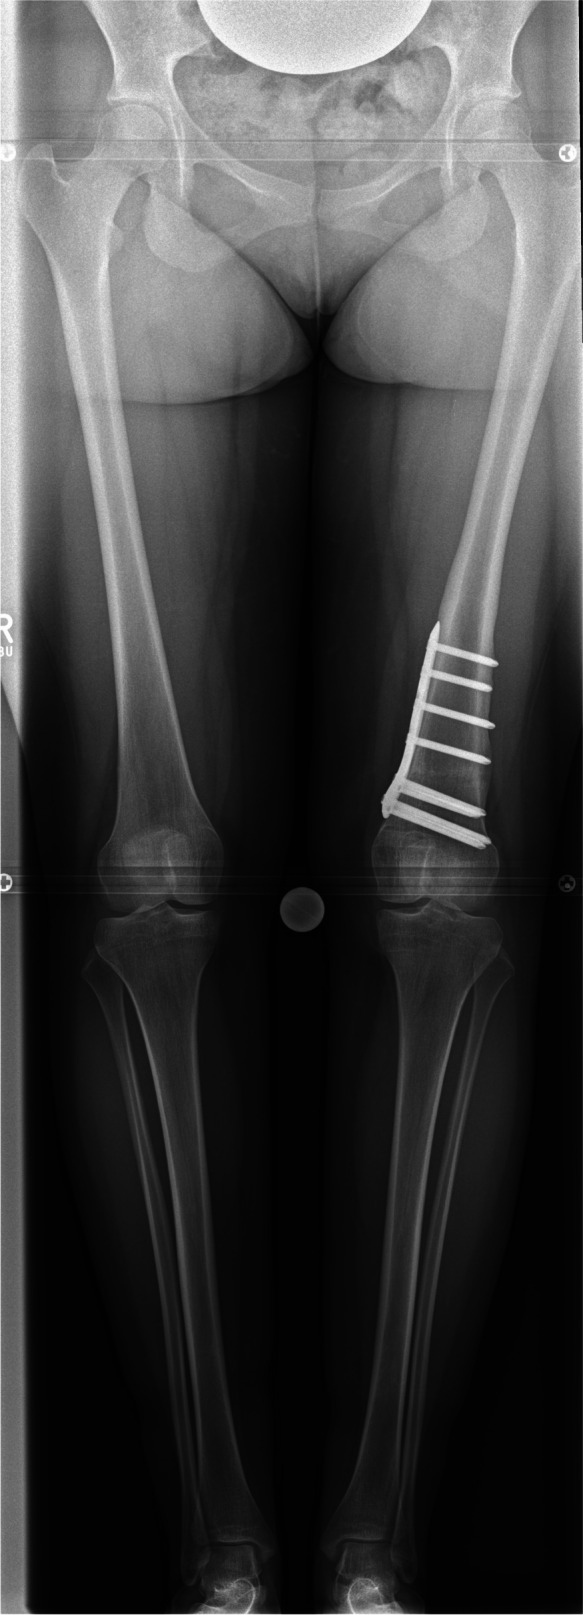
Antero-posterior long-leg weight-bearing radiograph after supracondylar derotation (= external rotation) osteotomy of the femur
